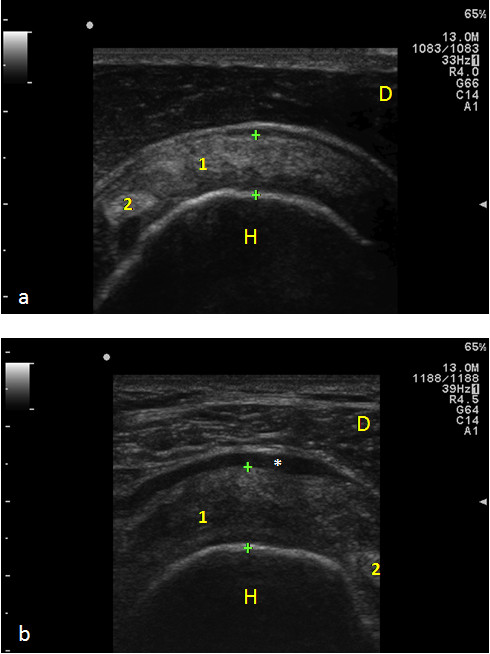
Ultrasound appearance of normal and degenerated Supraspinatus Tendon. Panel a) Transverse scan: a normal fibrillar pattern and thickness of Supraspinatus Tendon is observed (between calipers). Panel b) Supraspinatus Tendon is thickened (calipers), hypoechoic, dishomogeneous, with loss of the normal fibrillar pattern. In this picture, an effusion in the subacromial bursa is present (*). 1) Supraspinatus tendon; 2) Biceps tendon; H) Humeral head; D) Deltoid muscle.
